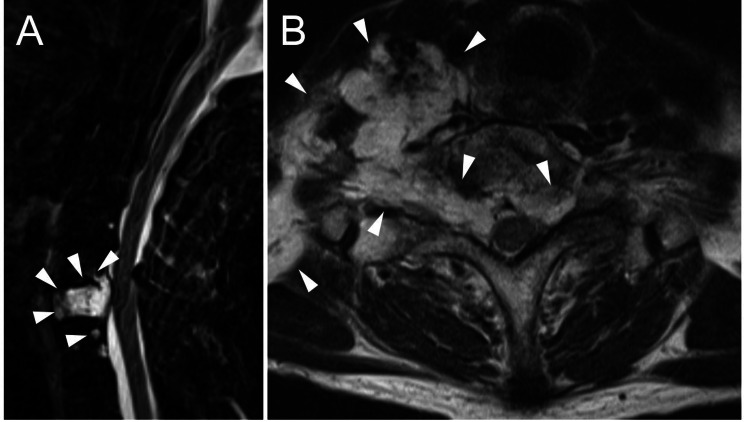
Cervical MRI with a STIR sequence and T2-weighted imaging before surgery (A) Cervical MRI with a STIR sequence showing a hyperintense lesion involving the C7 and T1 (arrowhead) vertebral body and compressing the spinal cord slightly. (B) T2-weighted axial imaging showing a hyperintense epidural tumor (arrowhead) involving both sides of the C7-T1 foramen. The tumor on the right side extends out of the foramen. MRI, magnetic resonance imaging; STIR, short inversion time inversion recovery.
